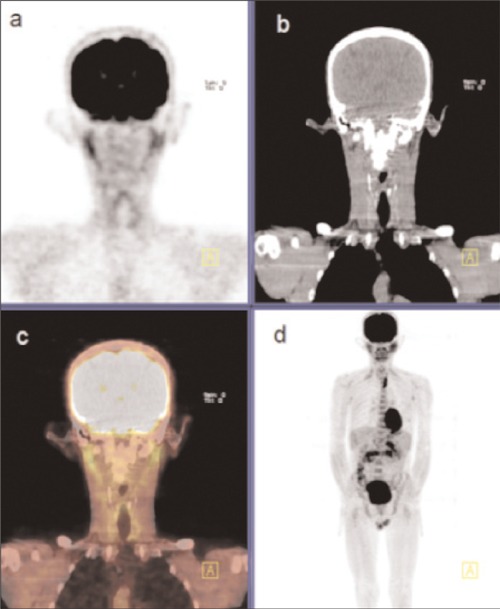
PET (a), CT (b), fusion (c) and MIP (d) images of the patientshowing unusual uptake at ears and nonspecific uptake at mediastinum
